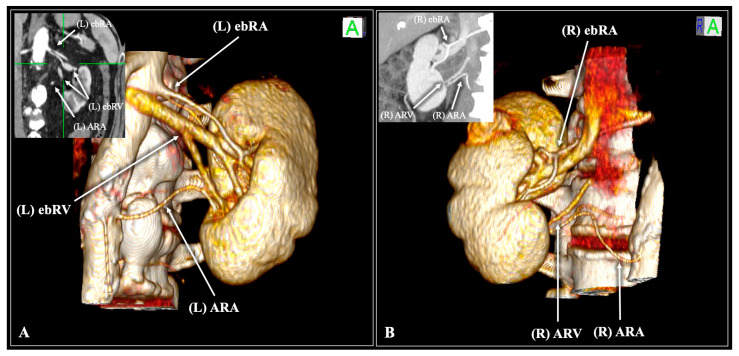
Contrast-enhanced computed tomography (CT) scan of a 61-year-old male (A) and a 68-year-old female patient (B) with three-dimensional and coronal reconstruction. The male patient (A) has an early bifurcated renal artery (ebRA) and vein (ebRV), as well as an accessory renal artery (ARA). The female patient (B) has an ebRA, an ARA, and an accessory renal vein (ARV). Note that the male patient (A) is demonstrating Artery (A)—Vein (V)—Vein (V)—Artery (A)—Pelvis (P)—Artery (A) variant. R—right, L—left, S—superior, I—inferior.
